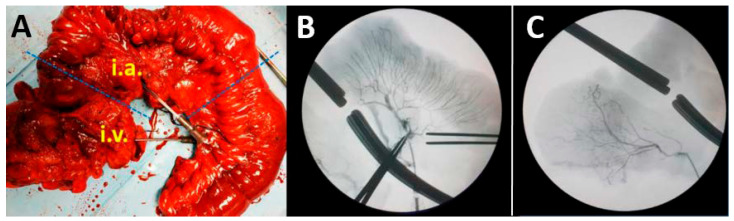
Example of arterial and venous vascularization of a colon segment affected by Crohn’s disease. The left panel shows a Crohn’s disease-affected colonic segment with catheters placed for gene injection (A). The middle and right panel are radiologic X-ray images showing tributary areas of a colonic artery (B) and a vein (C), respectively. Iodinated contrast solution was employed for its visualization. i.a.: intraarterial; i.v.: intravenous.
